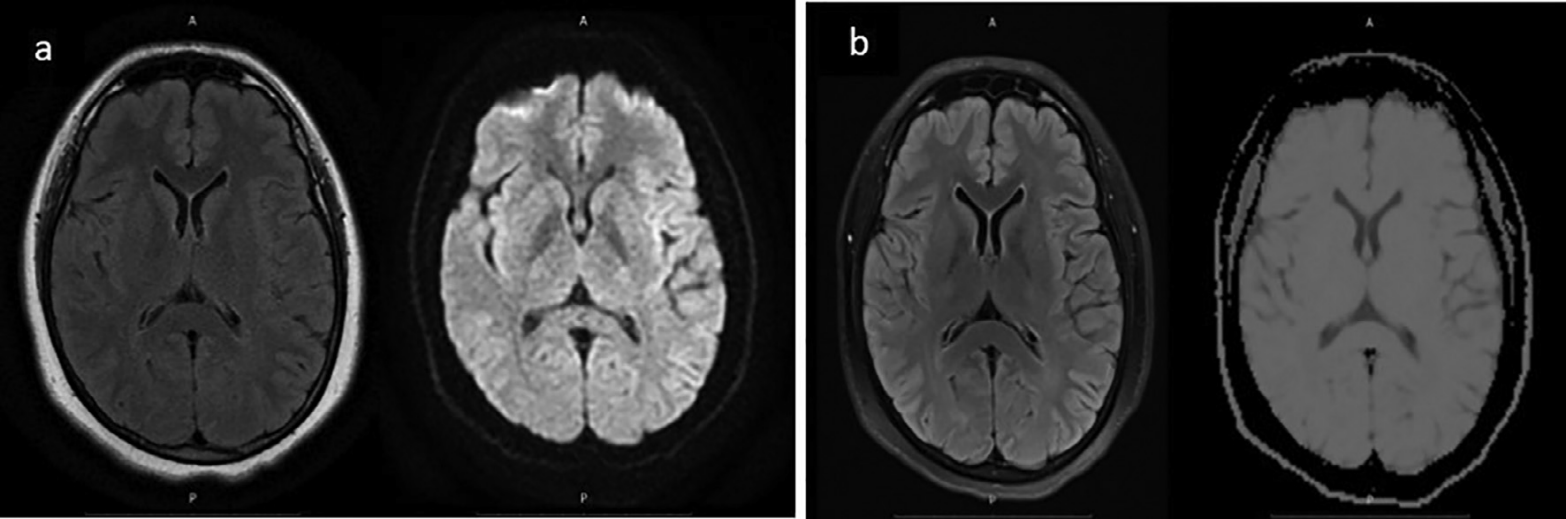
(a) Diffusion-weighted imaging (DWI)/Fluid-attenuated inversion recovery (FLAIR) normal (after the patient's second attack); (b) Normal DWI/FLAIR (after the third attack, 3 months after the first magnetic resonance imaging).
